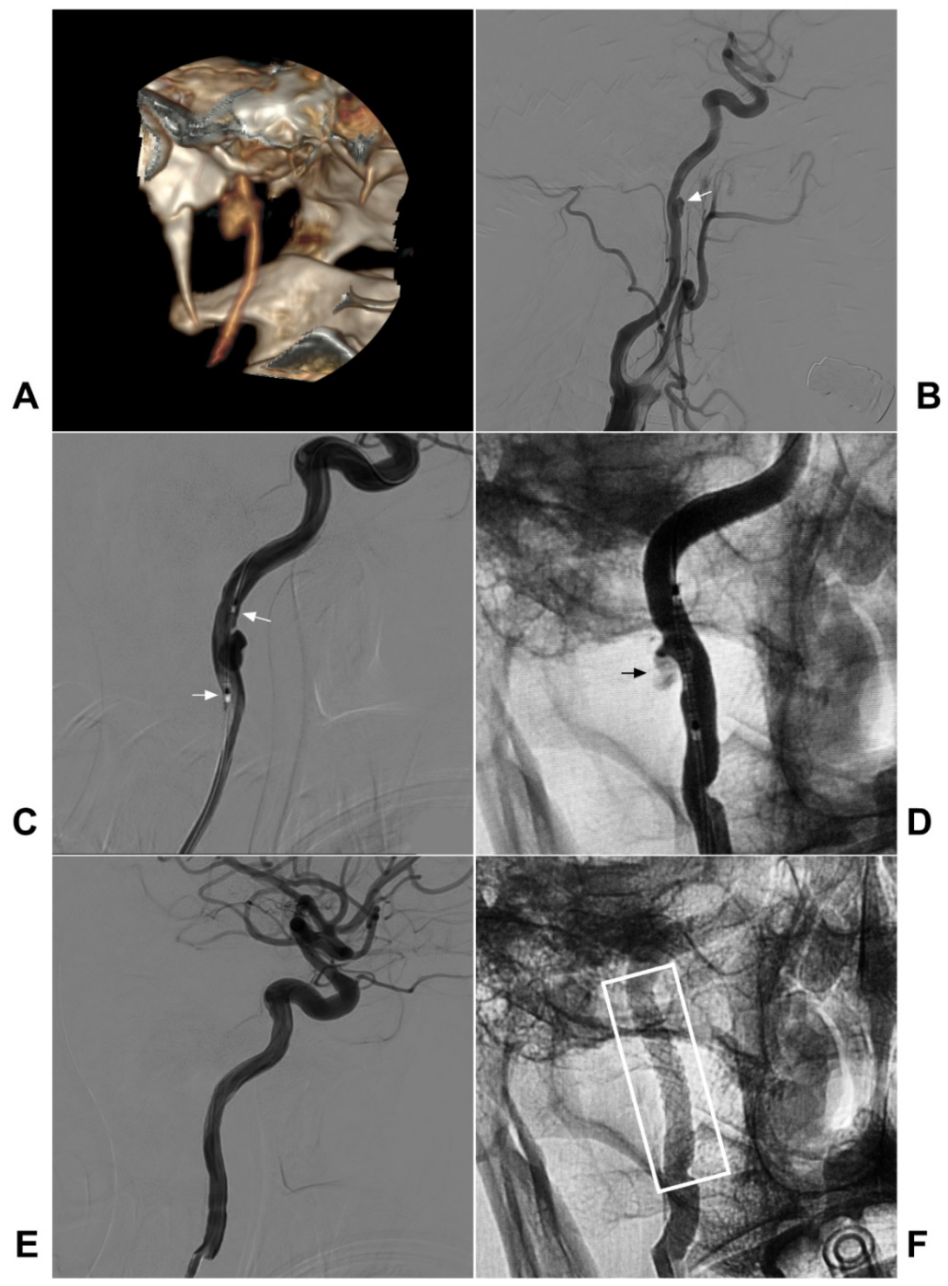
Covered stent deployment for the traumatic extracranial ICA pseudoaneurysm. A-B: CTA (A) and DSA (B) showed a pseudoaneurysm in the high segment extracranial CIA (arrow in B). C: A covered stent (MicroPort Medical Co., Shanghai, China) crossed the pseudoaneurysm (arrows show the distal and proximal markers). D: After covered stent deployment, endoleak was observable (arrow). E: After the balloon dilation was repeated, the endoleak disappeared. F: X-ray showing the covered stent (white frame). Abbreviations: CTA: CT angiography; DSA: digital subtraction angiography; ICA: internal carotid artery
